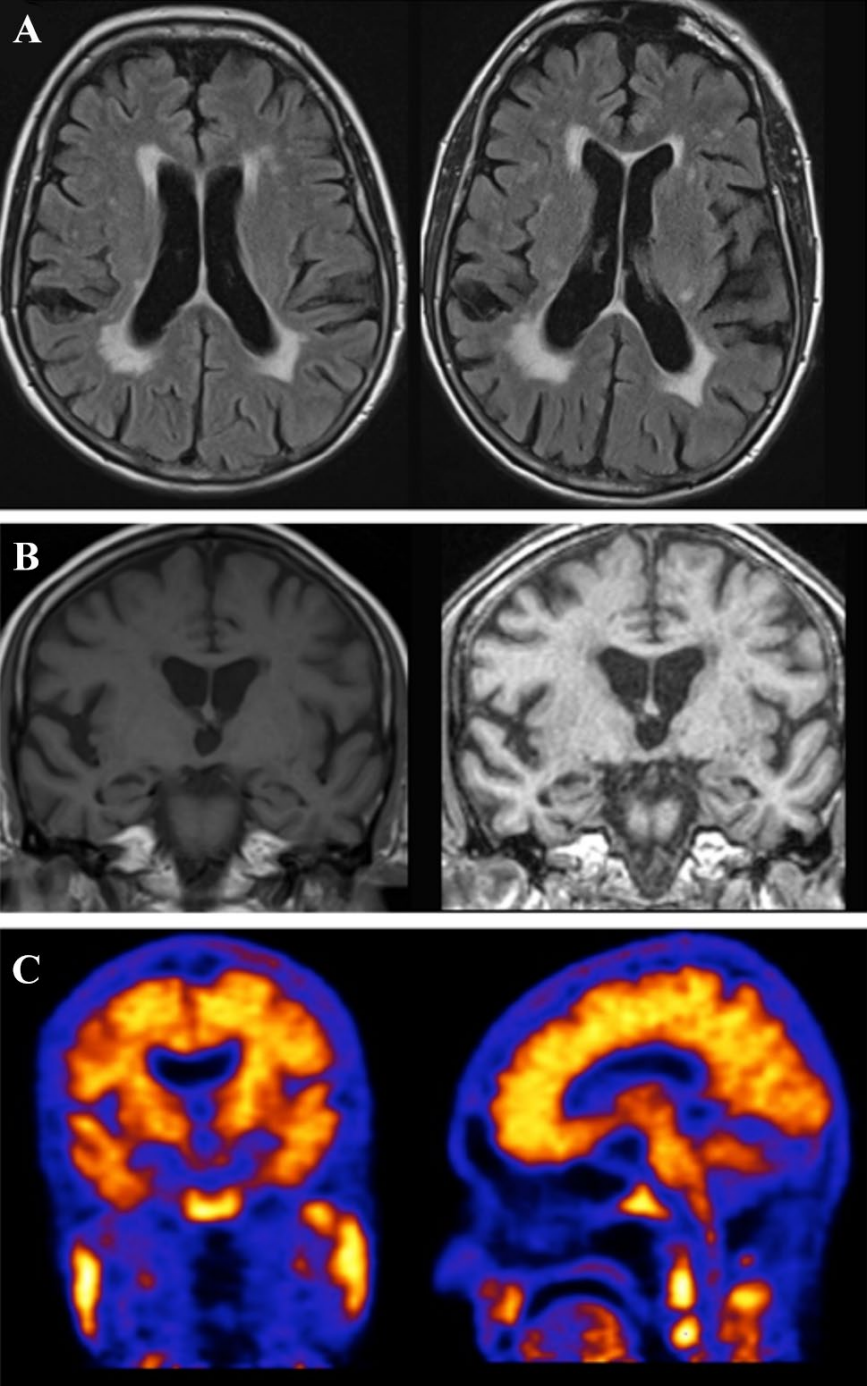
Case 3. Axial FLAIR images through the brain (a) demonstrating white matter changes over a 3-year interval. Coronal T1- and T2-weighted images through the brain (b) showing progressive atrophy of the brain over the 3-year interval. The most recent scan (right) shows bilateral medial temporal lobe atrophy, more so on the right. Amyloid PET imaging (c) was positive with loss of grey–white differentiation consistent with widespread amyloid deposition
